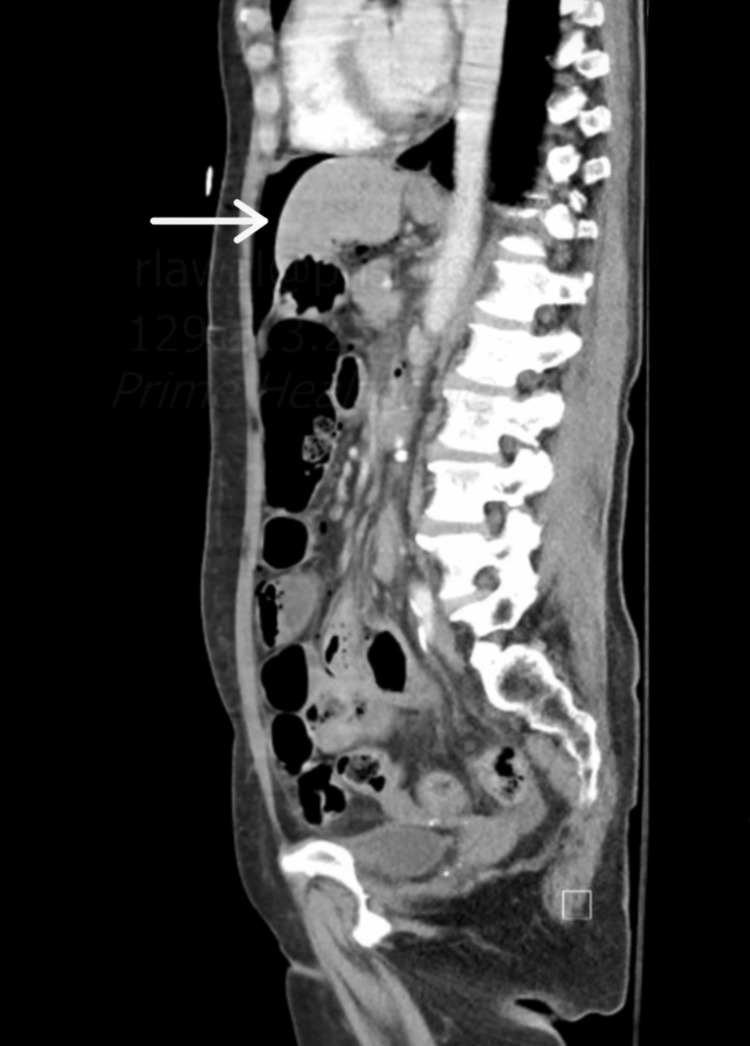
Sagittal CT image demonstrating pneumoperitoneum/free intraperitoneal air in the setting of hollow viscus perforation (arrow). CT: computed tomography
